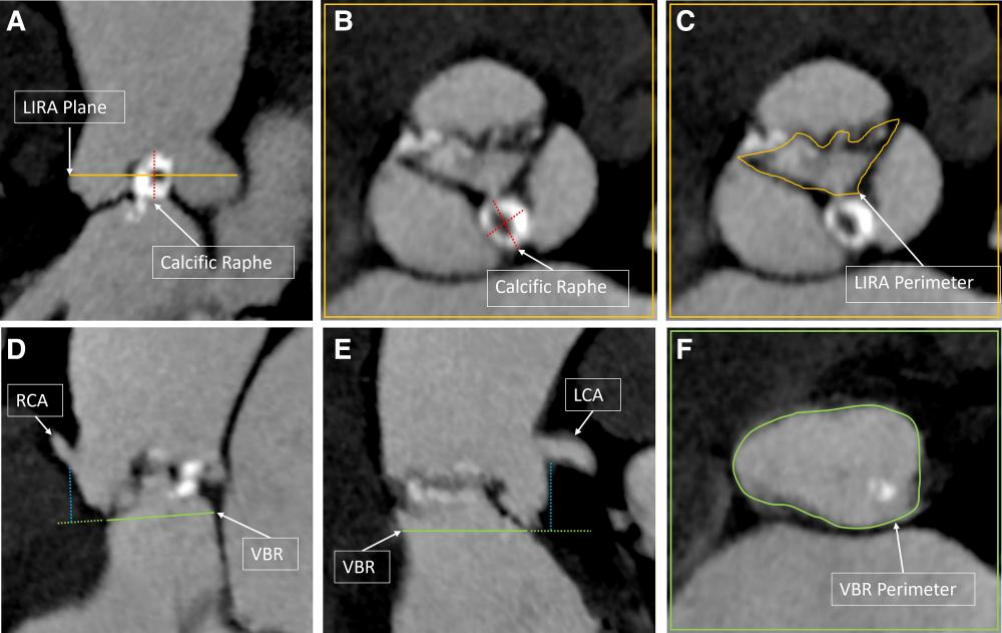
Pre-transcatheter aortic valve replacement aortic computed tomography scan. (*A* and *B*) Longitudinal and axial view at the raphe level (Level of Implantation at the RAphe plane, orange horizontal line, *B*) showing a bicuspid aorta root anatomy (Sievers Type 1) with a 9 × 7 mm calcific raphe (red dotted lines, *A* and *B*) between non-coronary cusp and left coronary cusp leaflets. (*C*) Prediction of the perimeter occupied by a transcatheter heart valve at the Level of Implantation at the RAphe according to the Level of Implantation at the RAphe method: Level of Implantation at the RAphe perimeter 82 mm (orange closed curve, *C*). (*D* and *E*) Longitudinal views at the virtual basal ring showing high coronary ostia (left 15 mm, right 14 mm) and wide Valsalva sinus. (*F*) Virtual basal ring measurements (green oval cuve, *F*: perimeter 92 mm, area 620 mm^2^), compatible with the largest available sizes of transcatheter heart valves. RCA, right coronary artery; LCA, left coronary artery; LIRA, Level of Implantation at the RAphe; VBR, virtual basal ring.
